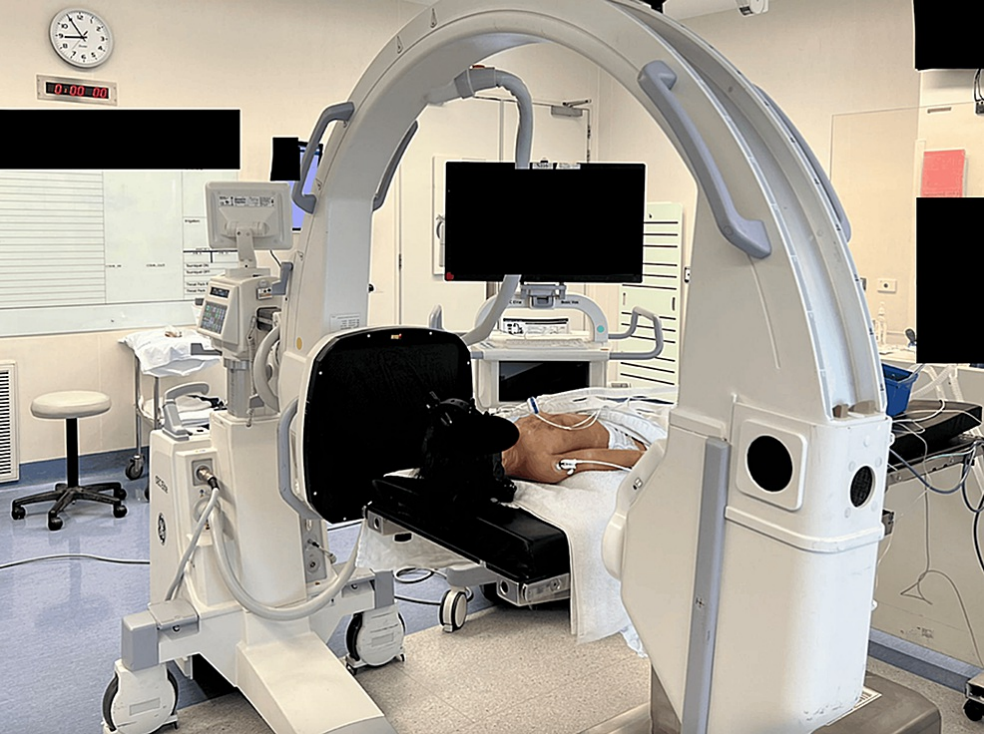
The C-arm is positioned for lateral X-rays. The monitor is positioned adjacent to the table so the surgeon has an unimpeded view of the screen during the examination (a patient from the case example).
